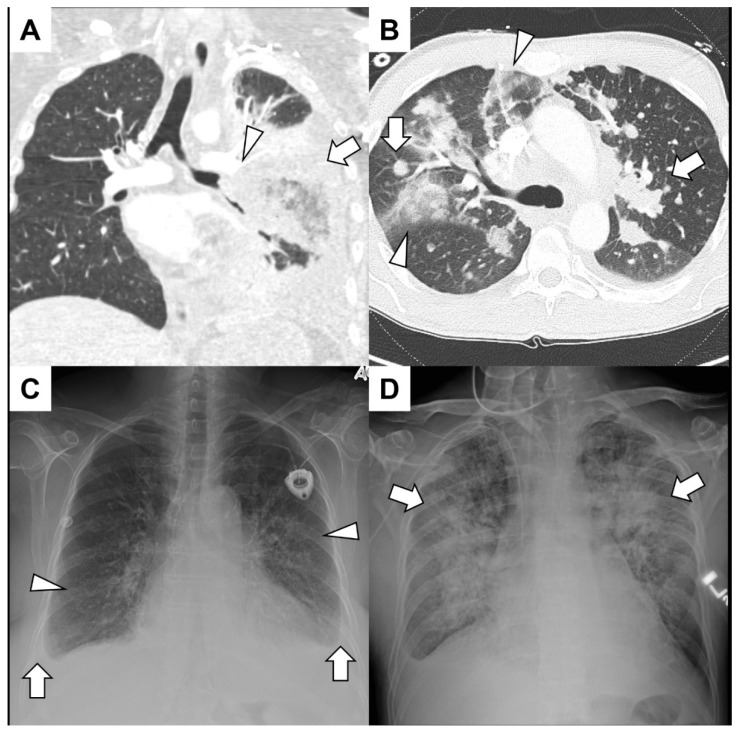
(A) A young man with acute lymphoid leukemia presented with significant hemoptysis. He had both neutropenia and lymphopenia due to recent treatments. A CT angiogram showed irregular soft-tissue encasement of the lower-order branches of the left pulmonary artery (arrowhead) and consolidation (arrow) and air bronchograms in the left lower lobe. Bronchoscopy revealed that the left lower lobe collapsed without endobronchial lesions. Surgical intervention with left lower lobectomy confirmed invasive mucormycosis and left pulmonary artery branches were full of thrombi. (B) A middle-aged man with adenoid cystic cancer presented with hemoptysis mixed with mucus over the previous few weeks. A CT angiogram excluded pulmonary emboli but showed diffuse nodules and mass-like consolidation (arrows) along with ground glass infiltrates peripherally (arrowhead). No endobronchial component was identified, and since the hemoptysis was of a small volume, no further intervention was warranted. (C) An elderly woman with a history of Hodgkin lymphoma presented with hemoptysis, dyspnea and a syncopal episode. A chest radiograph demonstrated bilateral infiltrates (arrowheads) and small bilateral effusion (arrows) with blunting of the costophrenic angles. She had volume overload with pulmonary edema, and an echocardiogram demonstrated severe aortic valve stenosis. (D) A patient with acute myelogenous leukemia after hematopoietic allogenic stem cell transplantation from a matched sibling presented with hemoptysis and epistaxis. A chest radiograph showed bilateral diffuse infiltrates (arrows), and a bronchoscopy revealed blood-tinged secretions throughout the tracheobronchial tree. He had diffuse alveolar hemorrhage due to thrombotic microangiopathy related to calcineurin inhibitors.
